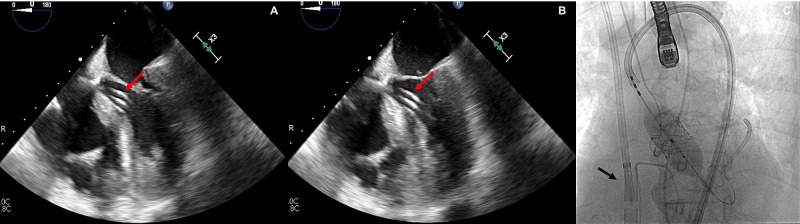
Intraprocedural transesophageal echocardiogram showing right ventricular global dysfunction with an Impella® left ventricular assistance device (red arrow) in the left ventricle. A) Diastole B) Systole. C) Fluoroscopy image depicting balloon valve inflation in-place with the extracorporeal membrane oxygenation system (ECMO) cannula in venous system (black arrow).
